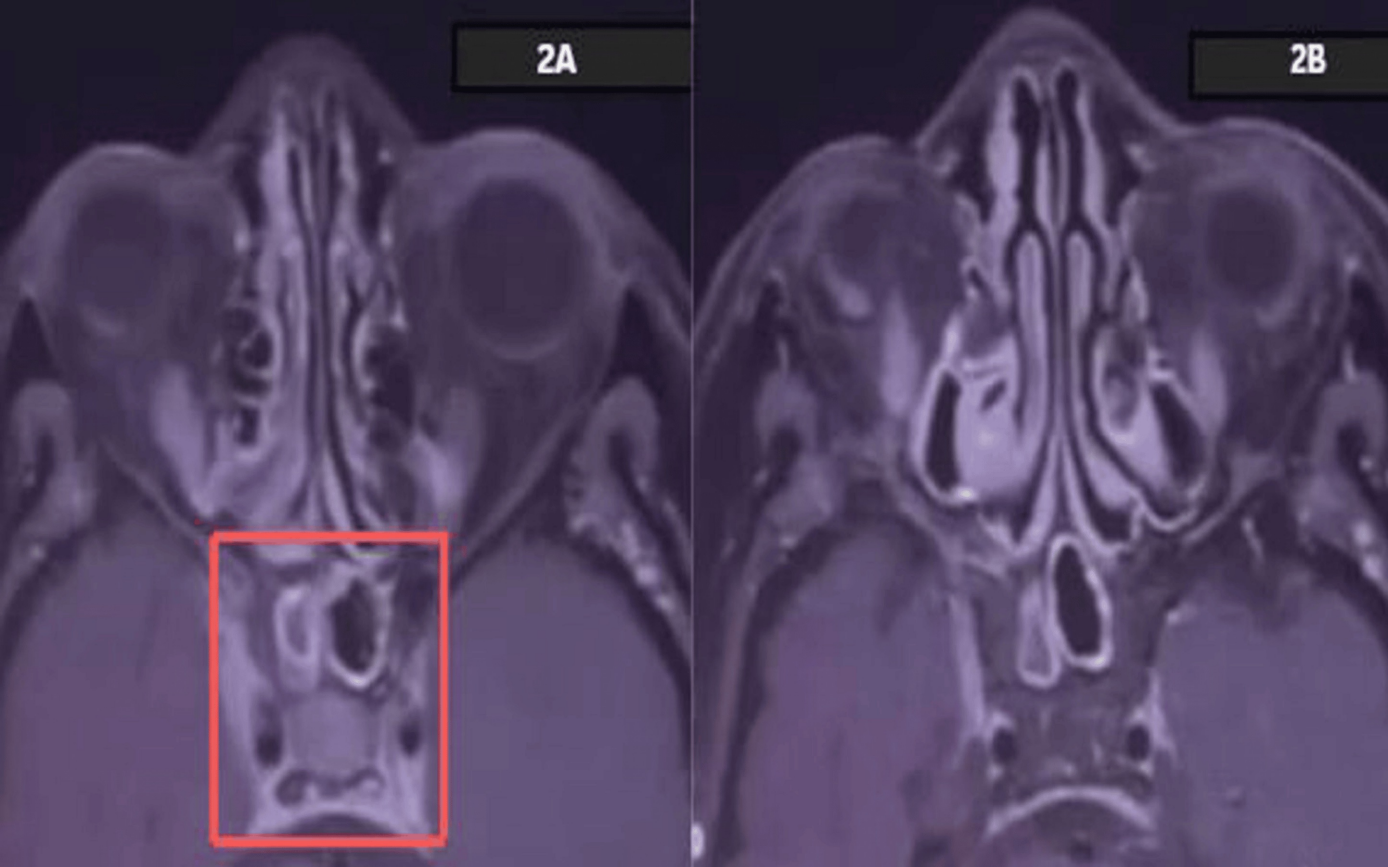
Axial MRI of the brain showing a hyperintense soft tissue lesion in the right cavernous sinus region 2A: MRI scan showing the coronal view of the nasal and paranasal structures. The image highlights the sinus cavities. 2B: MRI scan showing the coronal view of the nasal and paranasal structures with a slightly different contrast or setting, emphasizing the structural features in the sinus area.
